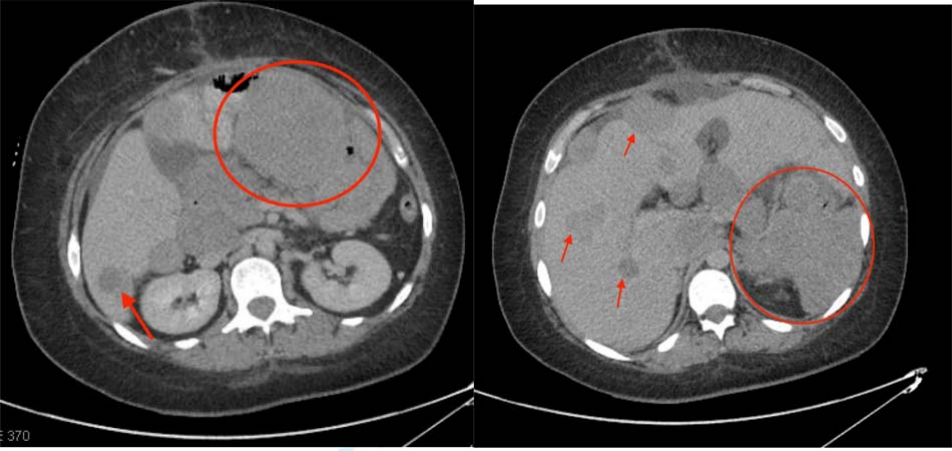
One-month post-operative follow-up CT scan showing progression of disease with large metastatic deposits (indicated by the red circles and arrows).
